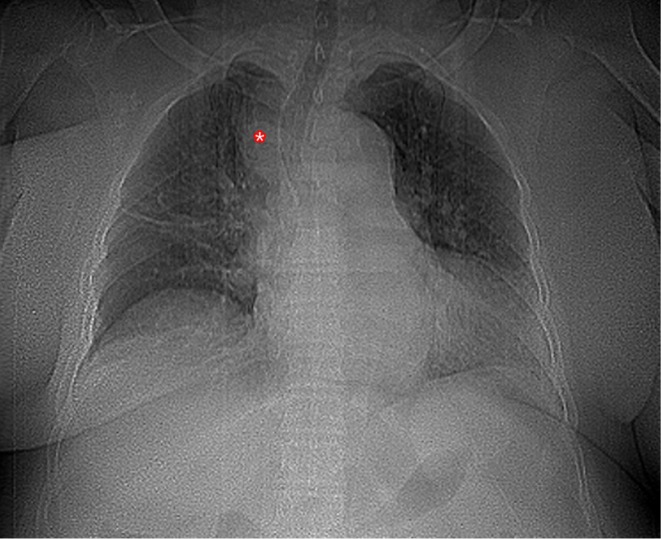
Scout view of CTA at the patient's initial presentation, reveals a smooth right paratracheal opacity (*) and dilated aortic arch.
